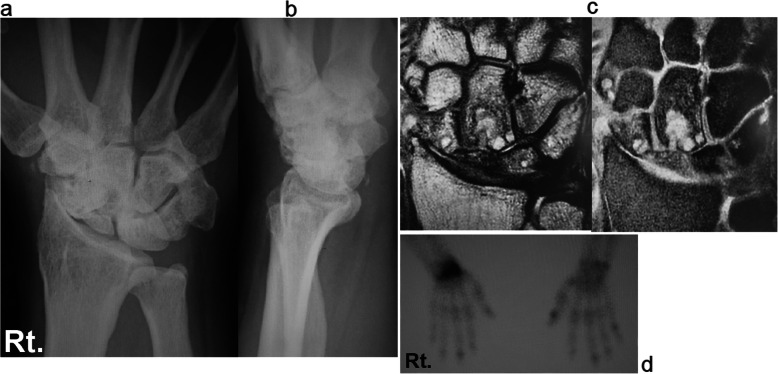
Radiographs (a, b), computed tomography scan (c), and bone scintigram (d) of a 56-year-old farmer. The patient suffered from radioscaphoid and capitolunate osteoarthritis of the right wrist caused by SNAC stage III following a fall 5 years ago. SNAC, scaphoid nonunion advanced collapse
